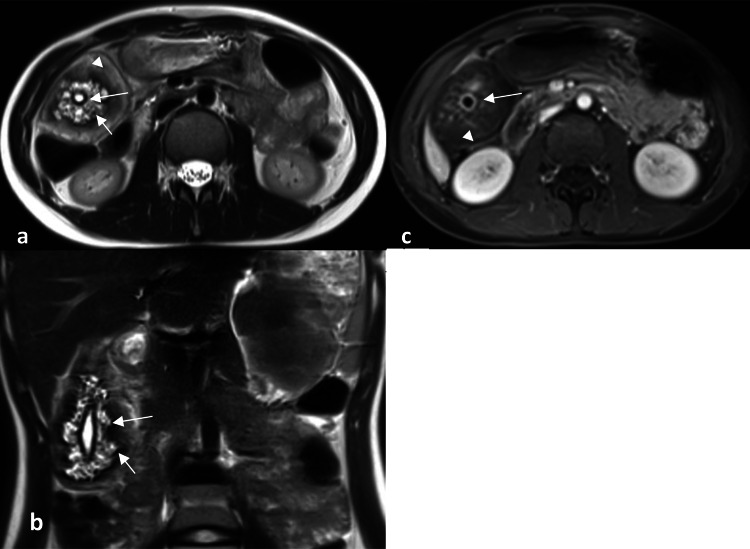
MRI images. MRI, T2W coronal (a), T2W sagittal (b), and T1W axial fat saturation with gadolinium (c). The gallbladder wall has five layers. The innermost zone is hyposignal T2W and enhancement on T1 fat-saturated (long arrow). The second layer has multiple cystic signals of RAS (short arrow). The third layer has hyposignal T2W enveloping the second layer of fibrosis. The fourth zone has hypersignal T2W and hyposignal T1W almost nonenhanced abdominal fat (arrowhead). The fifth outer area is hyposignal on both T1W and T2W of serosa signal. MRI: magnetic resonance imaging; RAS: Rokitansky-Aschoff sinuses; T1W: T1-weighted; T2W: T2-weighted
